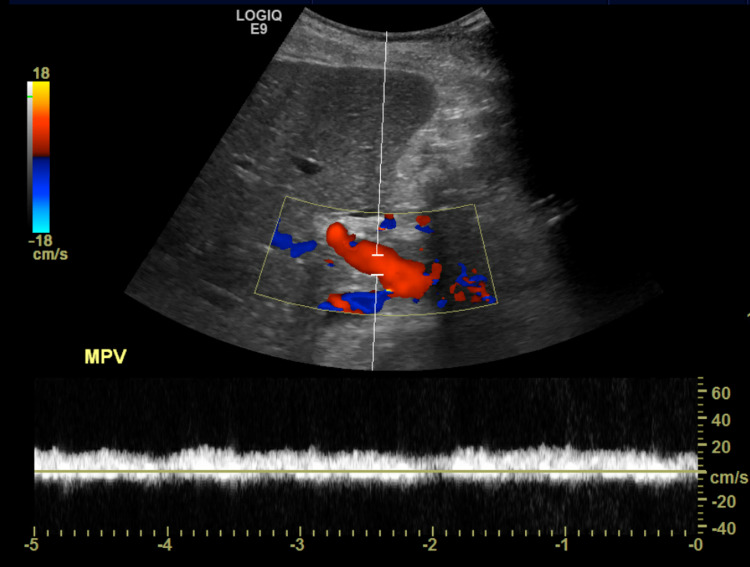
Doppler showing normal flow in the main portal vein. MPV: main portal vein.
